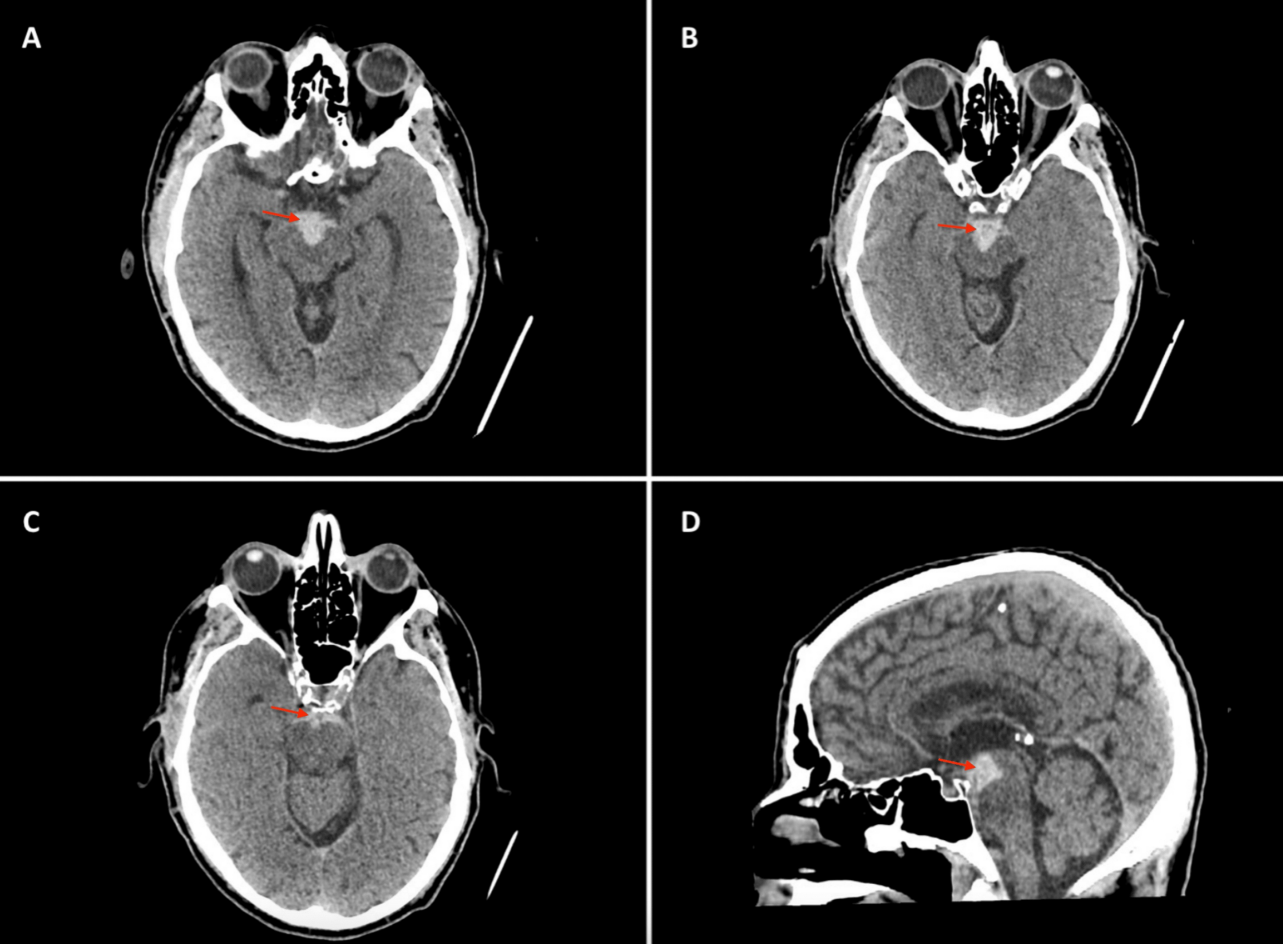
CT brain on initial admission. Arrows demonstrate localised acute subarachnoid haemorrhage within the pre-pontine and interpeduncular cisterns on axial (A-C) and sagittal (D) slices.
